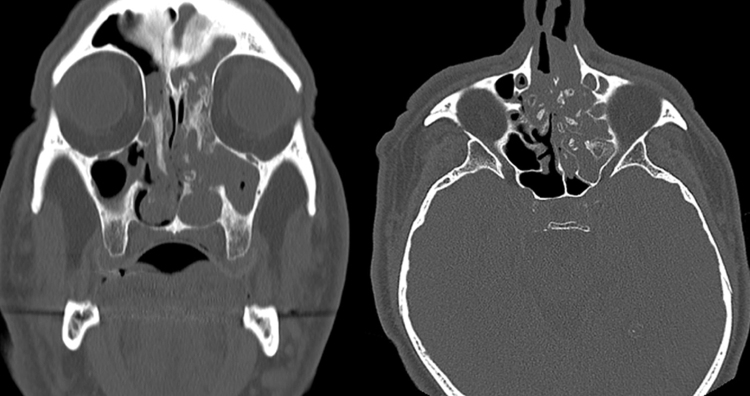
Bilateral multifocal osseous mass with soft tissue lesions axial and coronal CT images.
